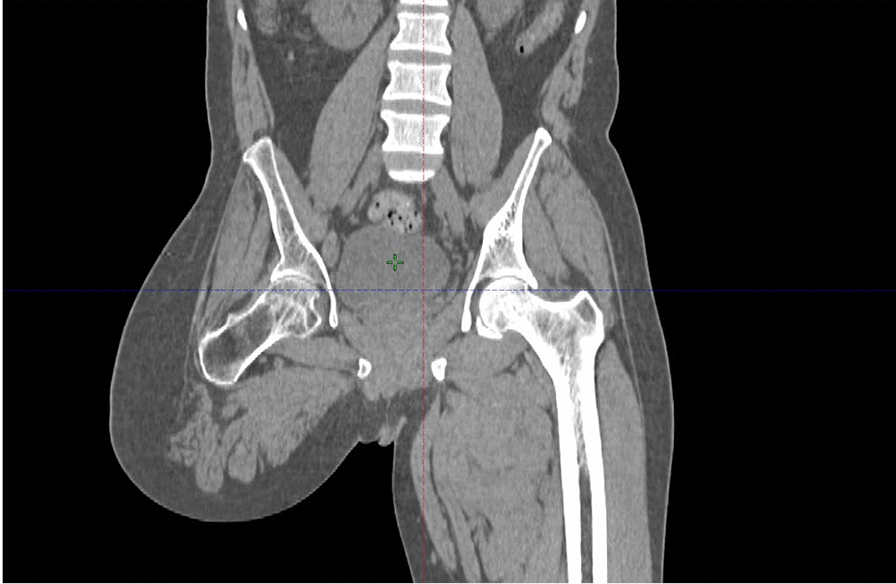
Coronal view of computed tomography scan of the pelvis showing transfemoral amputation
